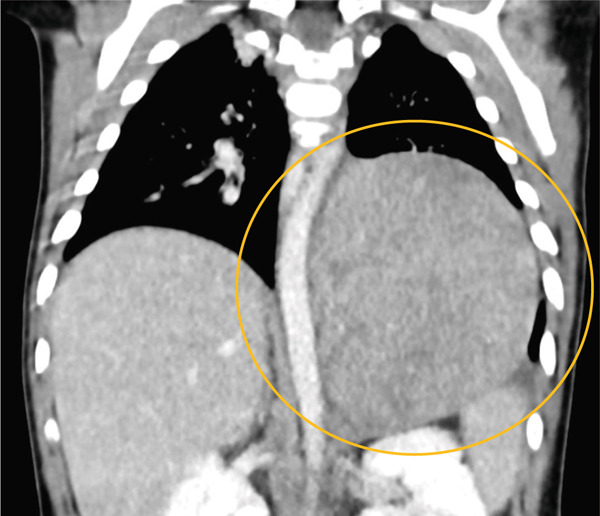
Coronal CT image revealing a large heterogeneously enhancing left intrathoracic/upper abdomen mass (yellow circle) with calcifications and superior extension compressing the left hemidiaphragm and adjacent lung parenchyma, resulting in lower lobe atelectasis.
